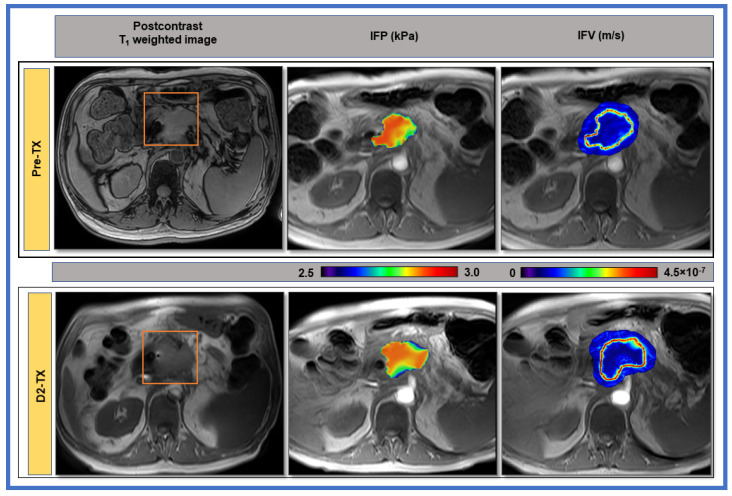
1st column: Representative pre- and post-stereotactic body radiation therapy (SBRT) T_1_-weighted post-contrast MR images of a patient (63 years, male) with PDAC shown in the body of the pancreas. The red rectangle delineates the region of interest. 2nd column: Computational fluid model estimates of interstitial fluid pressure (IFP). 3rd column: Interstitial fluid velocity (IFV) maps overlaid on pre-contrast T_1_-weighted images from PDAC patients at pre-and post-SBRT (D2-TX). A sharp drop in IFP at the tumor boundary results in evidently high IFV.
